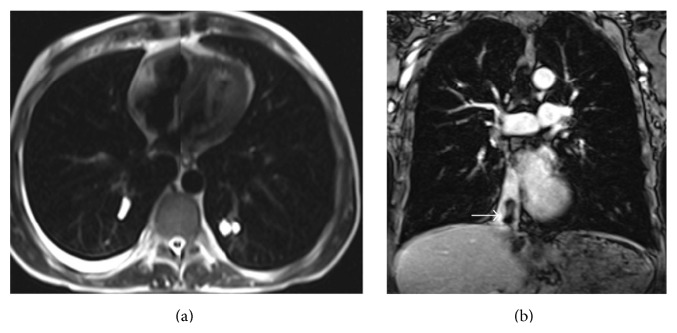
Axial T2-weighted MRI shows hyperintense cystic nodules in the lower lobe segmental pulmonary arteries bilaterally (a). Contrast-enhanced T1-weighted Vibe sequence coronal MRI shows contrast filling defect within the IVC due to ruptured liver hydatid cyst (b) (arrow).
